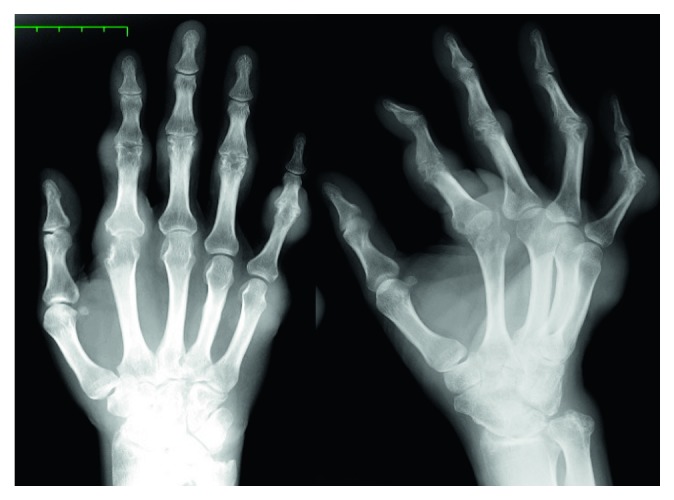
Preoperative radiograph of the right hand showing gouty arthritis and inflammatory osteolysis with numerous calcified soft tissue swellings, especially in the proximal interphalangeal joints of the index, middle, and little fingers.
